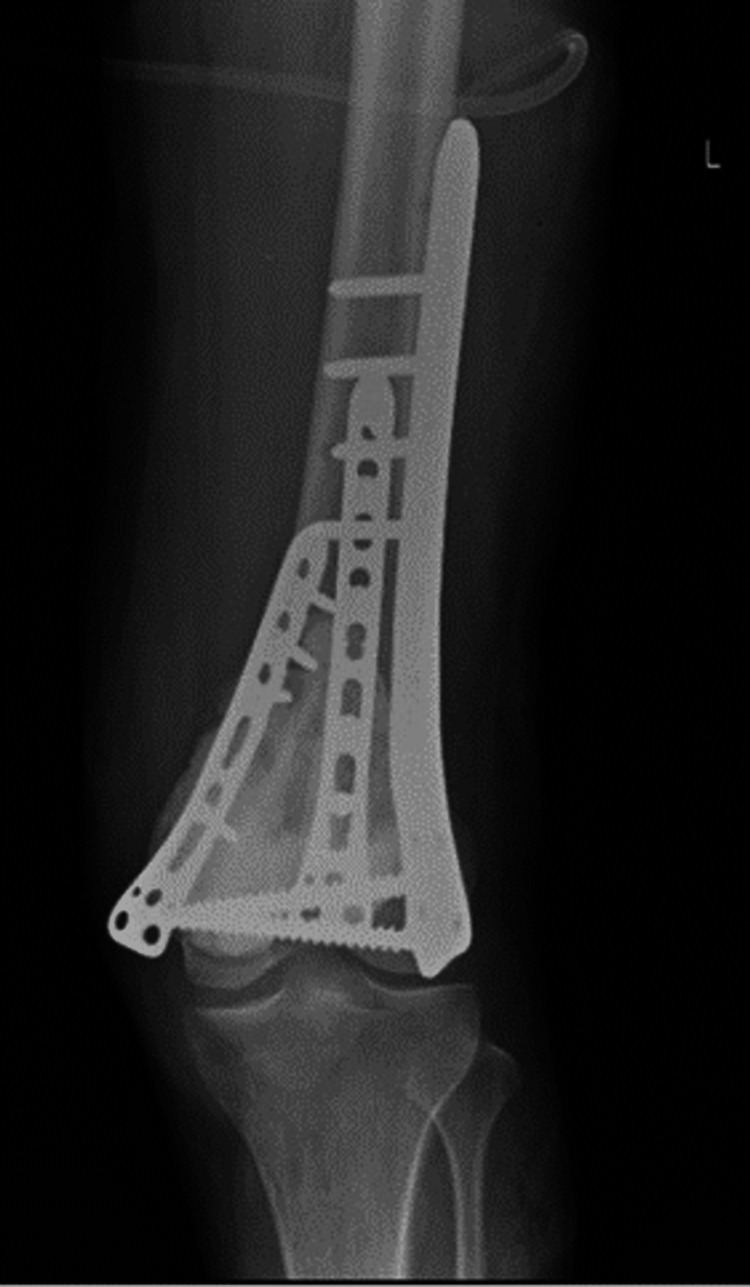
Postoperative plain radiograph, anteroposterior view of the left knee with 3-plate fixation
